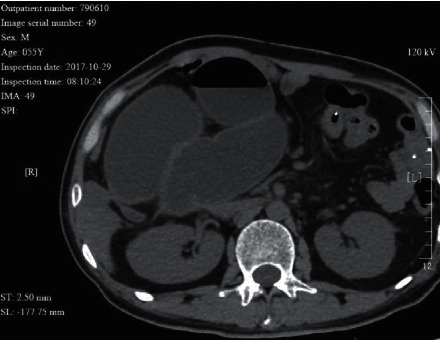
A 55 year-old male patient with adhesive intestinal obstruction. The CT image shows a closed loop. Bowel wall ischemia was seen during surgery.
